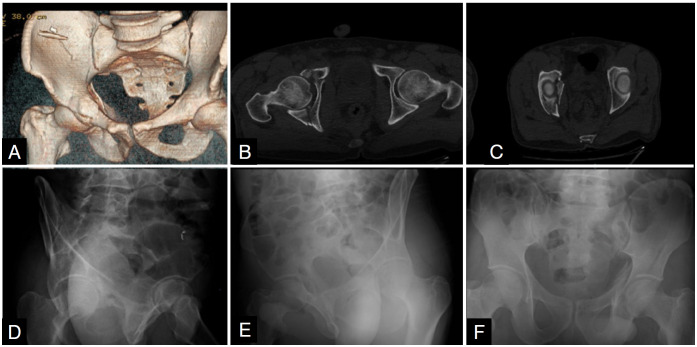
Patient eight radiograms; A: 3D-CT scan; B,C: 2D-CT scan; D: obturator oblique xray; E: iliac oblique xray; F: AP pelvis xray.
